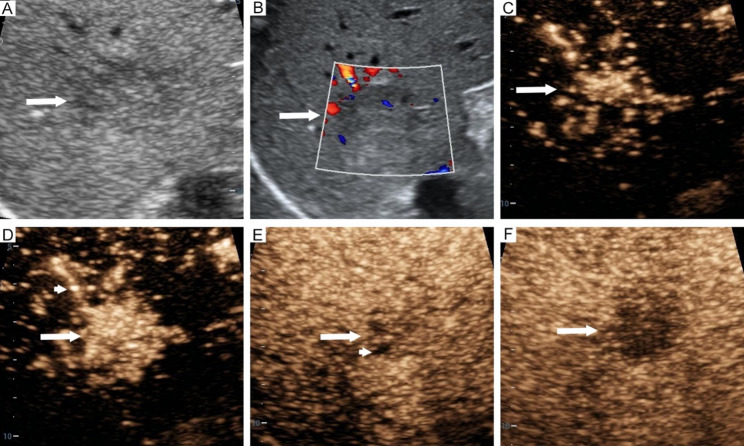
Imaging features of glypican-3-positive hepatocellular carcinoma in a 42-year-old man. Isoechoic lesion in segment 7 (a). Blood flow in the lesion (b). Contrast-enhanced ultrasound reveals early enhancement at 11s (c), feeding artery (arrowhead) at 13s (d), and necrosis (arrowhead) at 25s, all within the arterial phase (e), followed by hypo-enhancement at 94s in the portal late phase (f). Arrow indicates the hepatocellular carcinoma lesion
